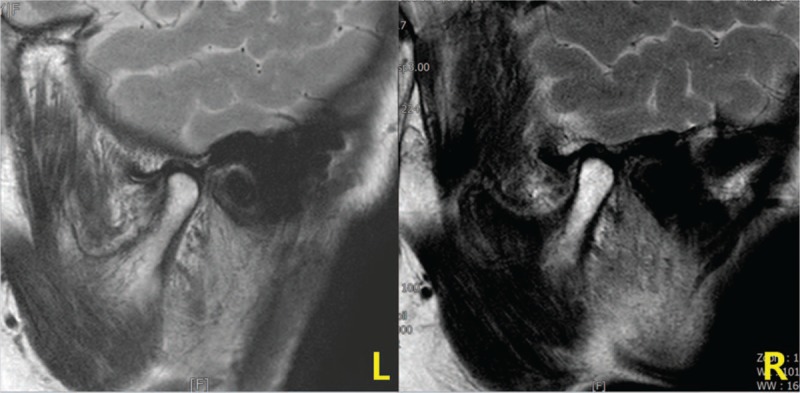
Temporomandibular joint (TMJ) magnetic resonance imaging (MRI). MRI showed anterior displacement of the left TMJ disc and mild anterior displacement of the right TMJ disc (April 17, 2017). L = left, R = right.
